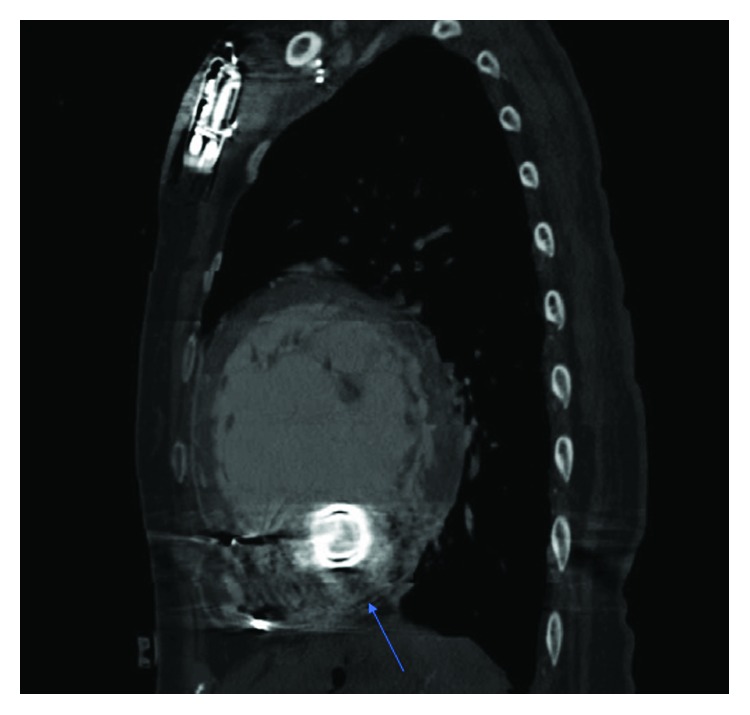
Gated CT scan of the chest with IV contrast in the sagittal plane demonstrating compression/thrombosis of the HVAD outflow graft (arrow).
